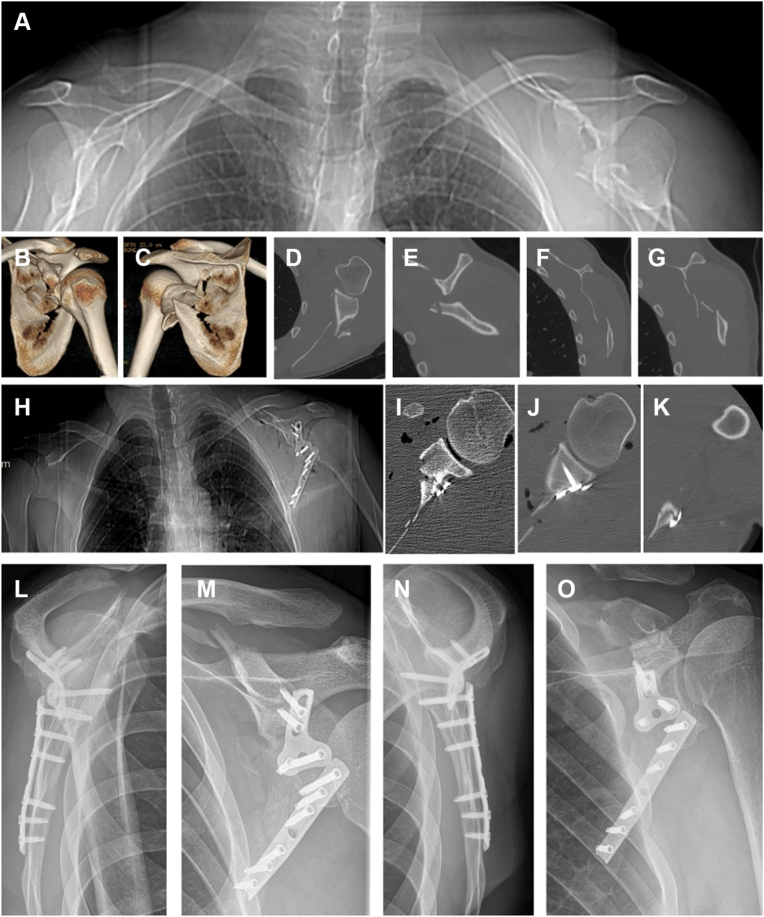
Double disruption injury of SSSC and its treatment. The injury occurred in a 29-year-old patient as a result of a sports-related trauma during a skiing accident. (A) Severely displaced scapular fracture involving the inferior displacement of the scapular body and coracoid process. (B and C) 3-dimensional CT image of the fracture. (D-G) Axial and coronal CT sections of the fracture. (H-K) Open reduction and internal fixation of the scapula using plate osteosynthesis. (L-M) Radiographs at 2-month follow-up, showing stable fixation and ongoing fracture healing. (N-O) Radiographs at 8-year follow-up, demonstrating full fracture union and maintained alignment without implant-related complications. A severely displaced scapular fracture with inferior displacement of the scapular body and coracoid process was treated with open reduction and internal fixation using plate osteosynthesis. Complete fracture union and maintained alignment were observed at 8-year follow-up without implant-related complications. These cases emphasize that double disruptions should be treated according to the unstable component of the ring rather than by a single universal fixation rule. Overall, the practical treatment principle for double disruptions is selective restoration of stability. Fixation of one dominant lesion may be sufficient when reduction of the remaining components is achieved indirectly and the SSSC ring becomes stable. Conversely, combined fixation should be considered when displacement persists, when the coracoid or acromion remains unstable, when glenoid or scapular neck involvement threatens shoulder mechanics, or when AC/CC instability prevents restoration of the clavicle–scapula relationship. Thus, the decision is not simply “operative versus nonoperative,” but rather whether the injured ring can maintain alignment and permit early functional rehabilitation without progressive deformity or chronic instability. *AC*, acromioclavicular; *CT*, computed tomography; *CC*, coracoclavicular; *SSSC*, superior shoulder suspensory complex.
